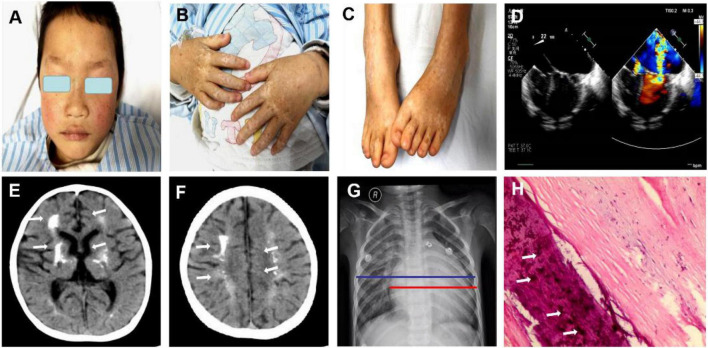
Clinical features of the patient with both dyschromatosis symmetrica hereditaria (DSH) and Aicardi-Goutières syndrome type 6 (AGS 6). The symmetrical appearance of freckle-like pigmentation and hypopigmentation spots were on the face (A), backs of hands and feet (B,C), presenting a reticulate pattern. Enlarged cardiac shadow was observed through Chest radiographs, and the cardio-thoracic ratio was around 0.65. (D) Cardiac color ultrasound showed normal postoperative cardiac changes after patent ductus arteriosus closure, severe mitral regurgitation (MR) and moderate mitral stenosis (MS), extremely mild aortic regurgitation (AR), mild + tricuspid regurgitation (TR) and a small amount of pericardial effusion (PE). (E,F) Brain computed tomography revealed calcification in the dentate nucleus, basal ganglia and cerebral white matter and mild brain atrophy. The textures of both lungs were increased and blurred, and there were small patchy shadows (G). (H) A mitral valve biopsy revealed significant valve calcification, and the arrows marked the calcification.
